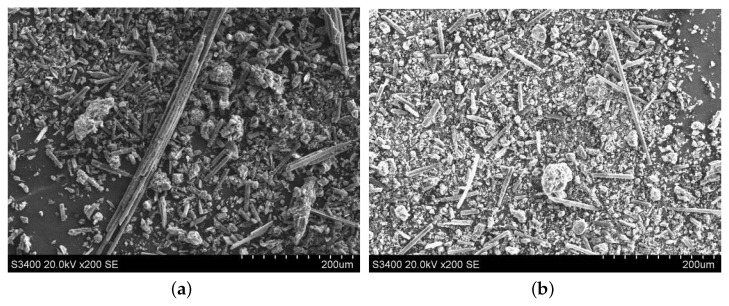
Morphologies comparison when $a_{p}$ is 0.3 mm. (a) is ordinary cutting and (b) is ultrasound- assisted cutting.
